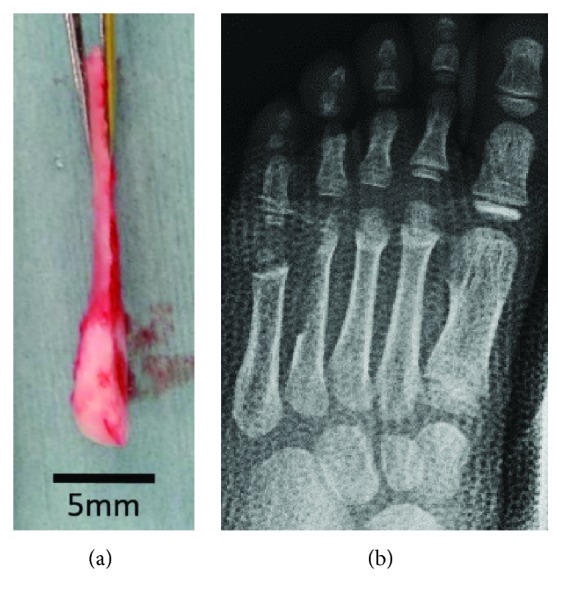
The metatarsal bone cut hemilongitudinally: (a) the cut metatarsal bone and (b) radiograph after the removal of the fourth metatarsal bone.
